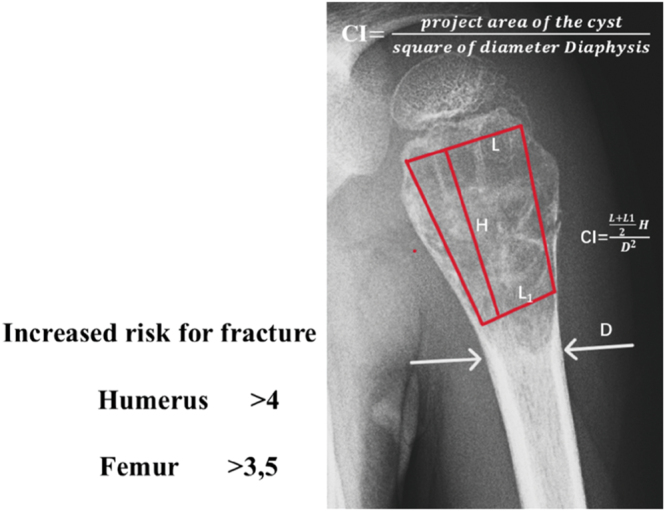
Kaelin & MacEwen found that the larger the cyst, the more cortex was destroyed and the bone was weakened. To quantify the strength of the remaining cortex, which depends on the size of the cyst and the size of the affected bone, they developed the cyst index. This indicates the relationship between the radiographic area of the cyst and the size of the affected bone, measured as the square of the diameter of the diaphysis (19).
